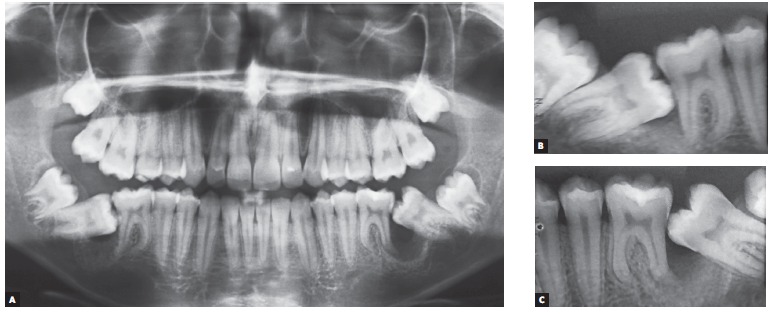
Initial radiographic aspect: panoramic radiograph (A) and periapical radiographs of teeth #46 and #47 (B), and #36 and #37 (C).
